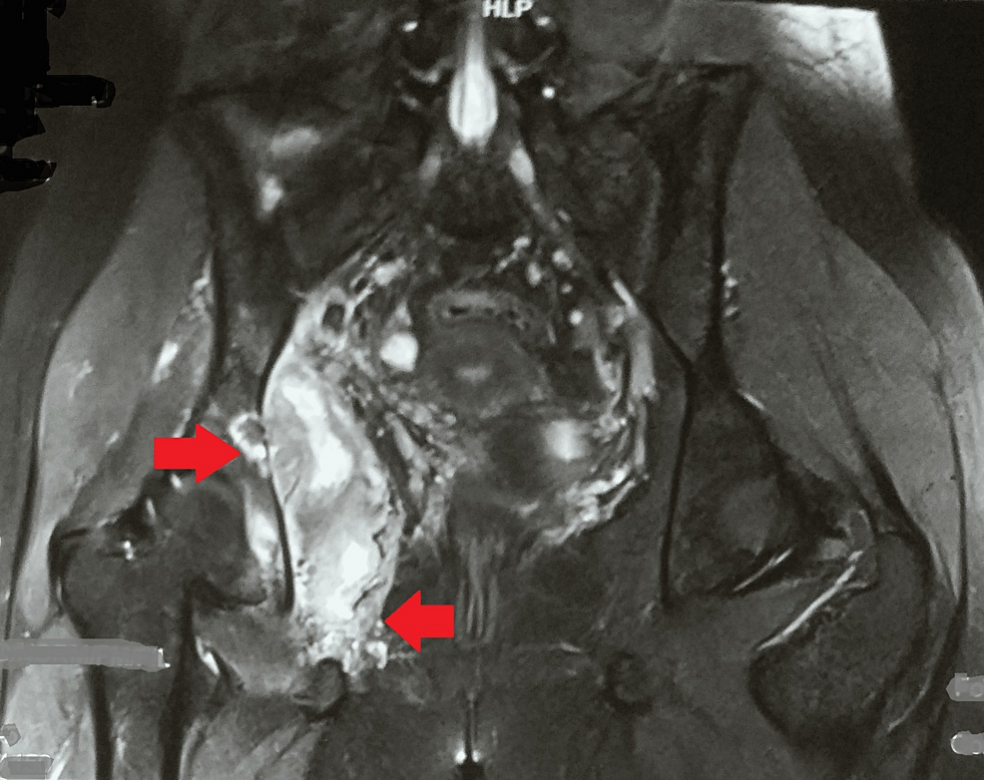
MRI of the right hip joint showing a large multiloculated intercommunicating collection/abscess in the right iliopsoas muscles, obturator internus, and obturator externus MRI: magnetic resonance imaging
